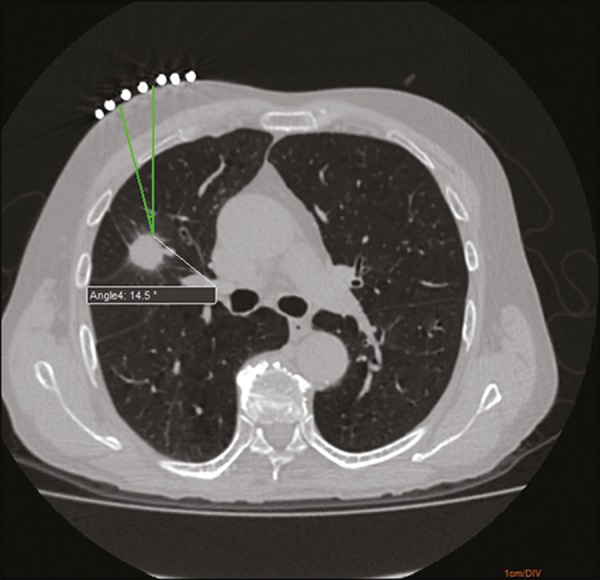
Axial CT of the chest, showing the puncture site planning, with angle
